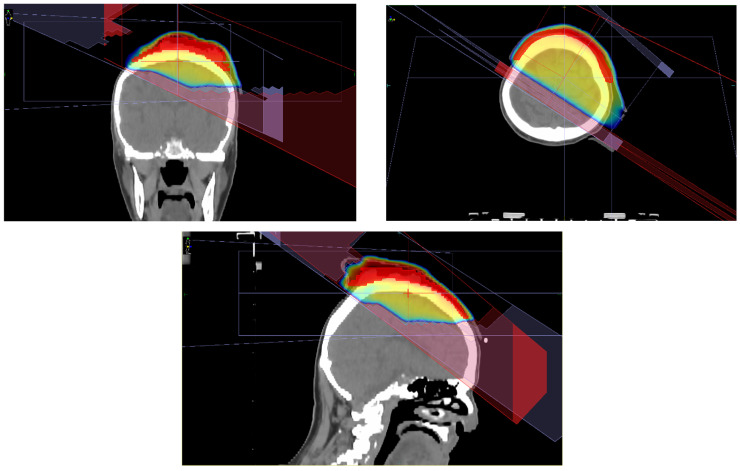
Representative ultra-high frequency ultrasound-driven modification of radiotherapy target delineation.
